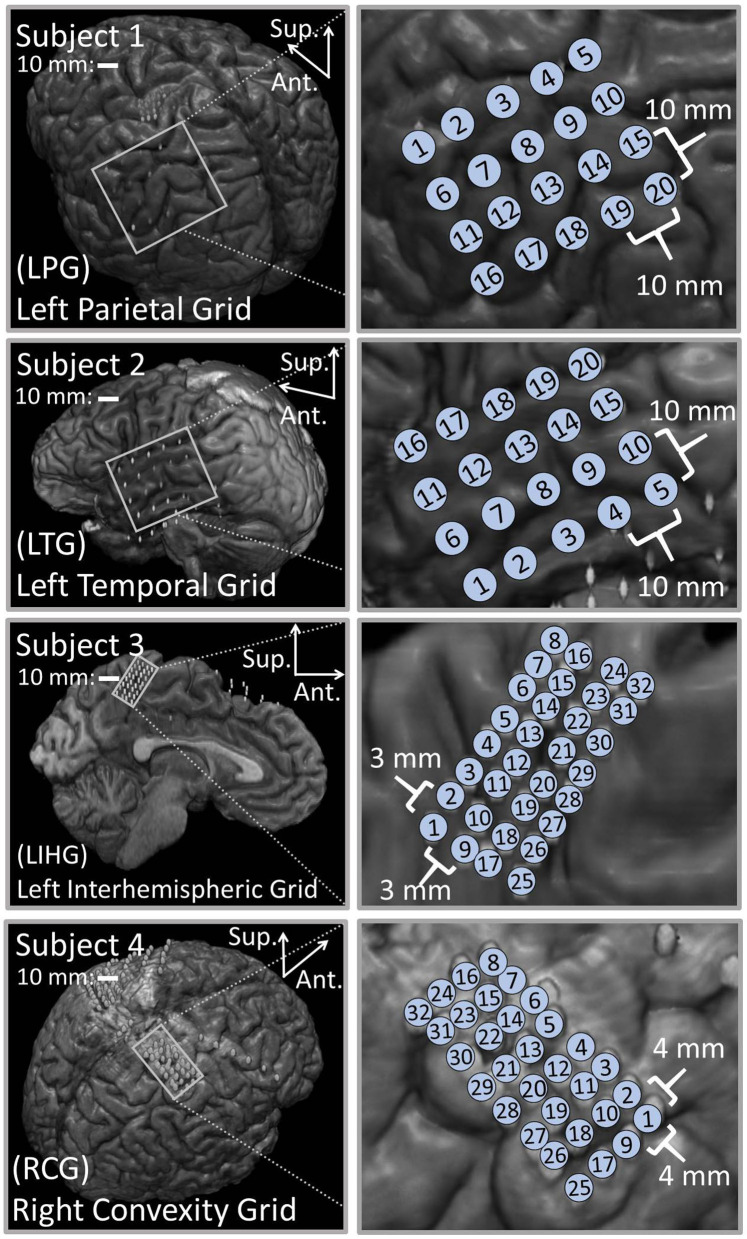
Co-registration of electrodes segmented from CT images and brain segmented from MRI. (Left) Co-registration image for each subject, with the representative grid outlined in white. Note that the points representing electrodes are not to scale. Also note that the co-registration image for Subject 3 shows a left hemisphere (sagittal plane) with electrodes in the inter-hemispheric fissure. (Right) Insets of representative grids with electrode numbers encircled. The label size is not related to the electrode size.
